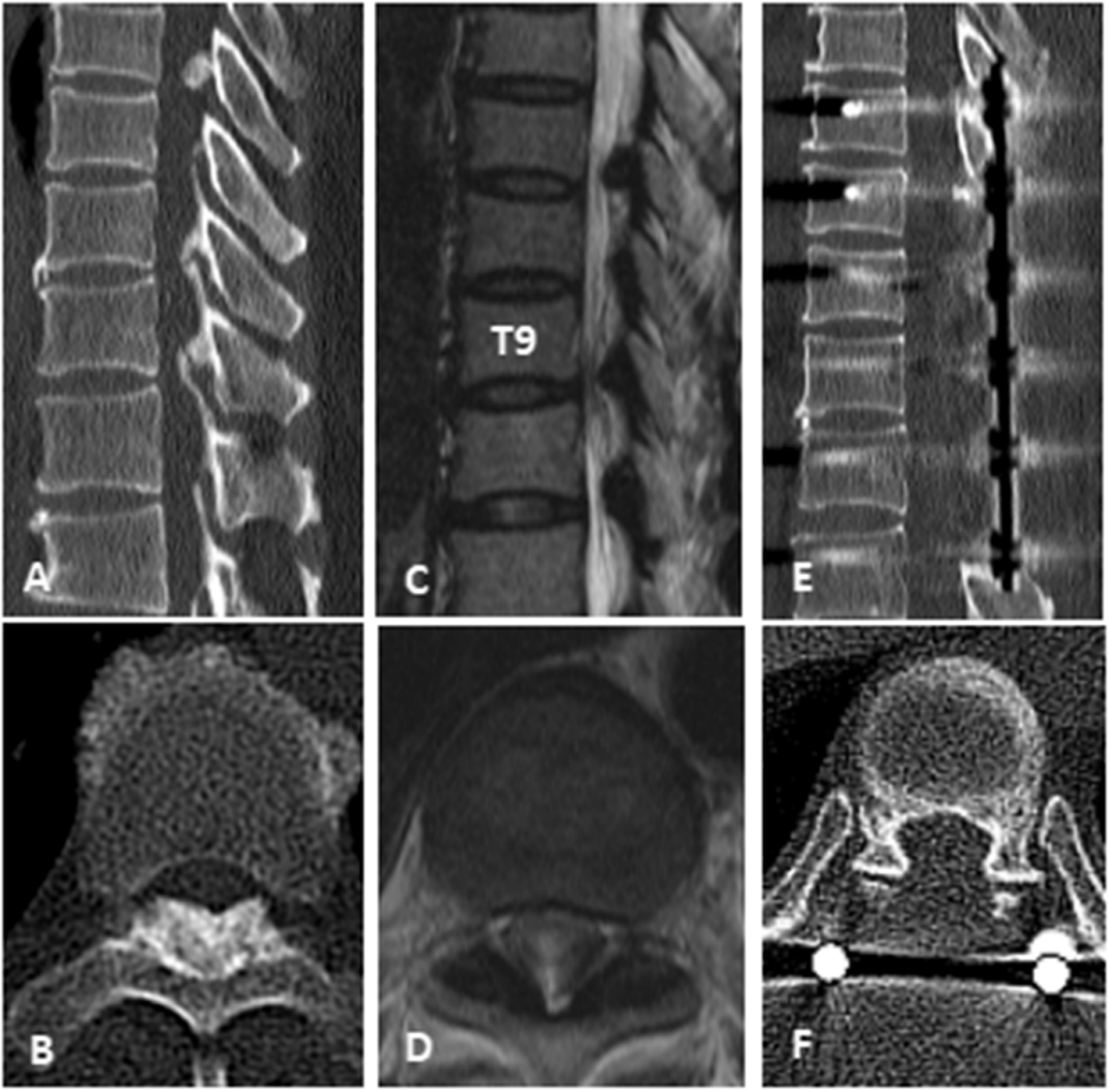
Laminectomy with instrumentation. A 62-year-old male patient, ossification of ligamentum flavum and compression degeneration of spinal cord were seen in CT (figure A, B) and MRI (figure C, D) before operation. We performed OLF resection with LI (Fig. E, F). During the 2-year follow-up, the preoperative JOA score increased from 4 to 8 (JOA score improvement rate was 57.1 %)
